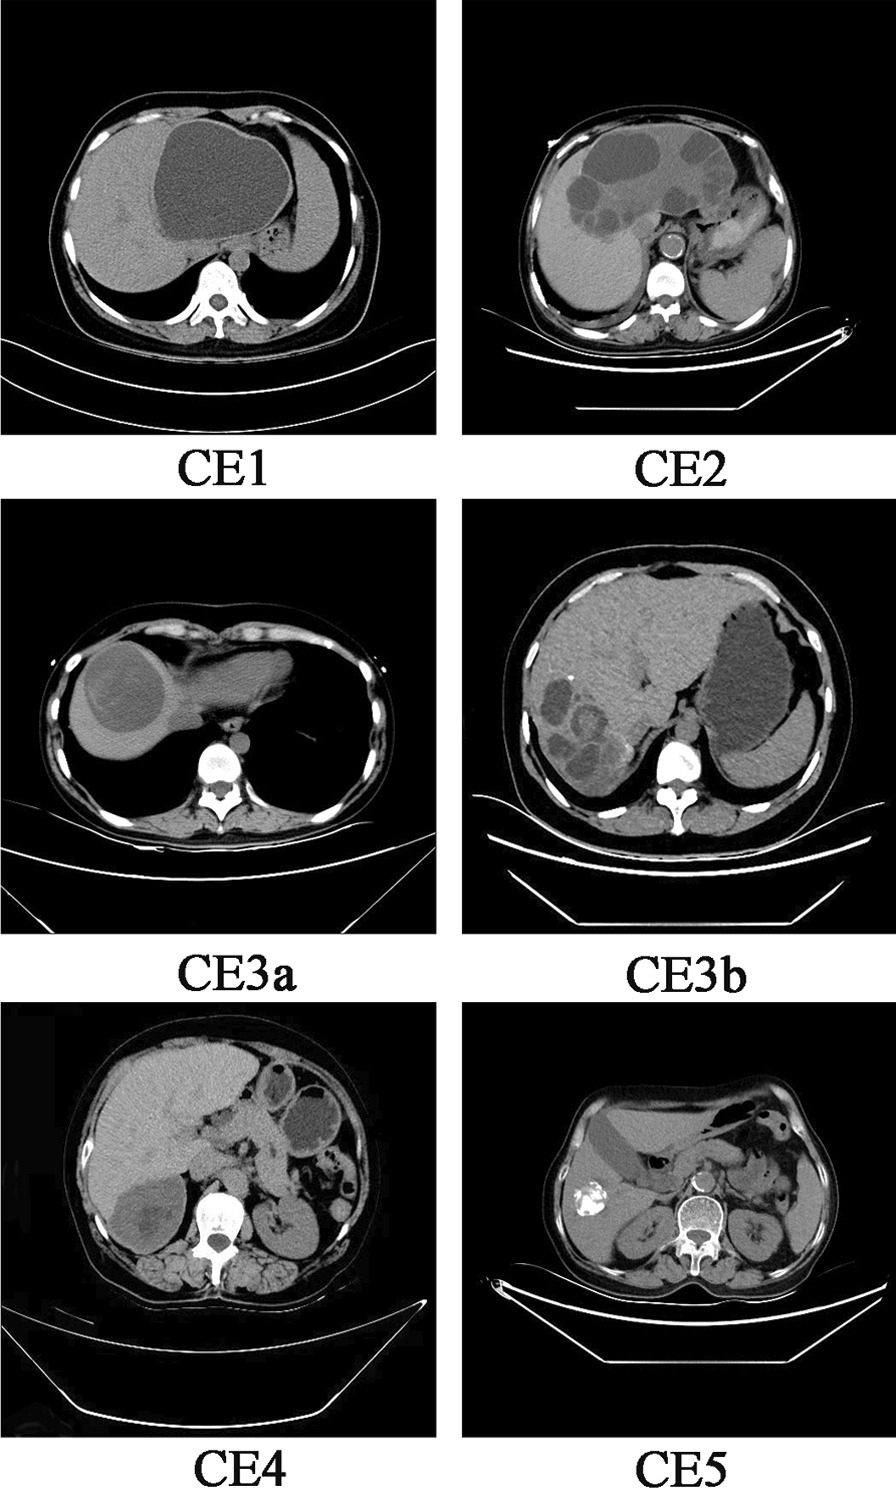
CT images of different type of hydatid cysts in patients from Ningxia Hui Autonomous Region, northwest China. CE1: unilocular cyst with liquid content, CE2: multivesicular, multiseptated cysts, the cysts are well delineated, smooth with uniform content, CE3a: cysts with liquid content and detached endocyst, CE3b: cysts with daughter cysts inside a mucinous or solid cyst matrix, CE4: heterogenous solid cysts with degenerative content and CE5: cysts with degenerative content and heavily calcified wall
